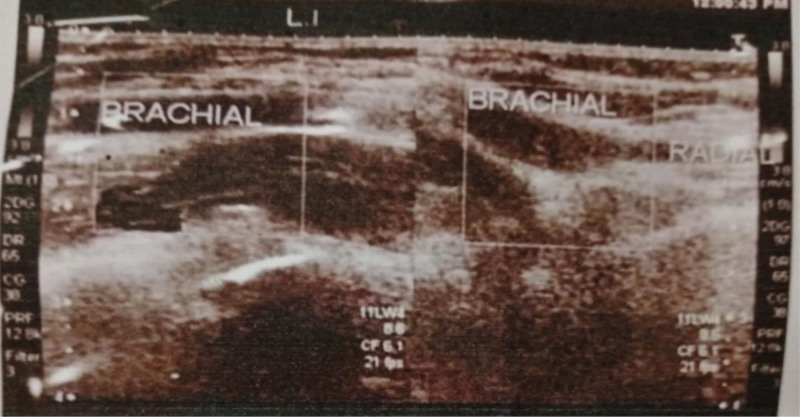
Doppler ultrasound of the left hand brachial artery indicated significant atherosclerotic changes, along with echogenic material seen in the left distal brachial artery extending into its branches (radial and ulnar arteries), completely occluding blood flow.
